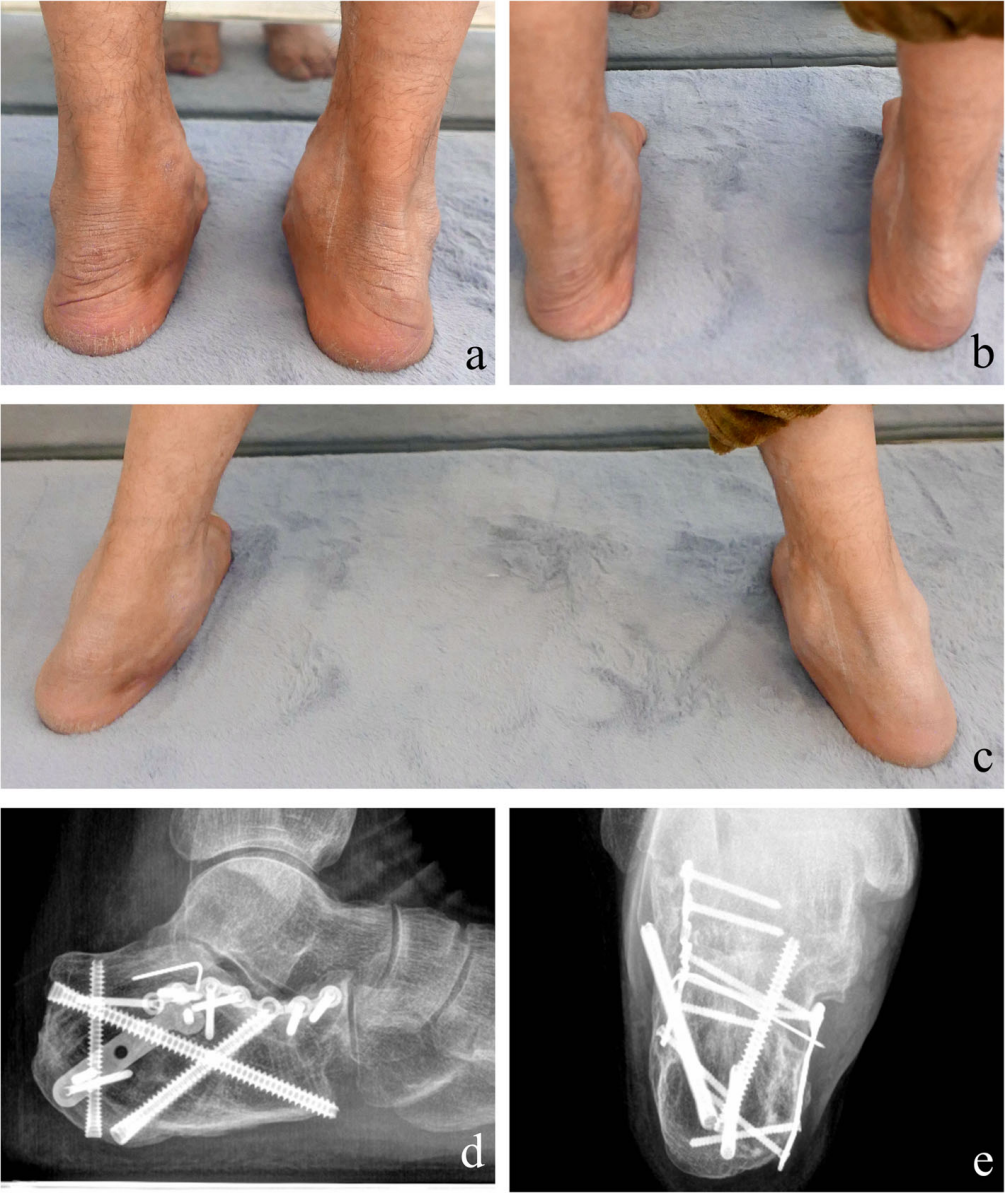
Radiographs of the patient in Fig. 1 at 40 months after surgery. a–c Postoperative view shows that the functional have returned to near-normal. d Calcaneal lateral axis view shows good reduction of the fractures. Bohler angle, 25°; Gissane angle, 125°; calcaneal height, 47.30 mm; calcaneal length, 77.64 mm. e The calcaneal width was decreased after surgery. Calcaneal width, 40.04 mm
